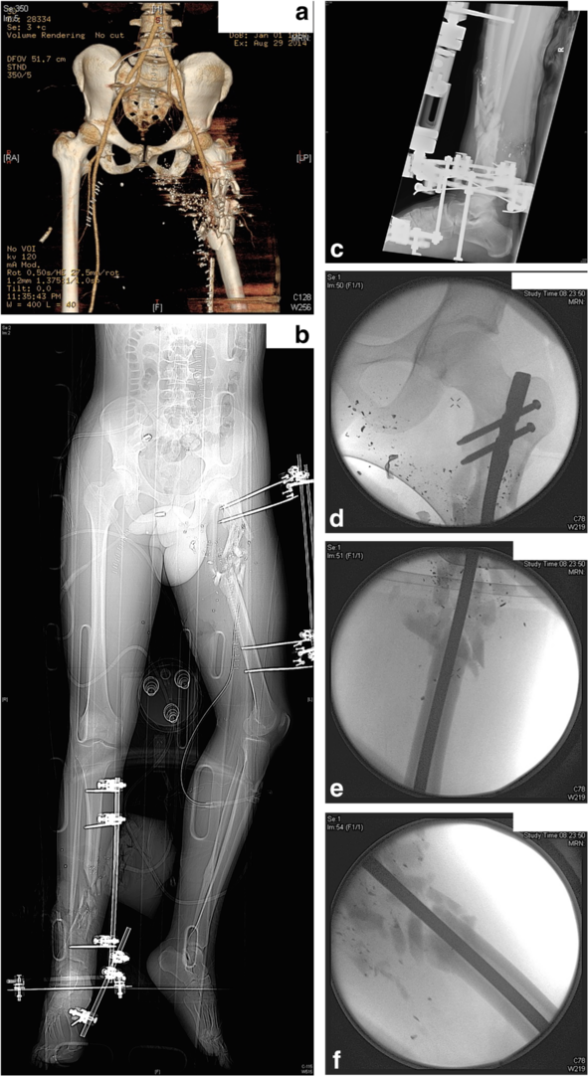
a 3D reconstruction showing extent of left comminuted open proximal femur injury. b Scout radiograph showing the original DCO procedures to the right tibia and left femur as described in the text. c Post-operative view of the right tibia after conversion to a hybrid ring fixator. d-f Intra-operative radiographs showing the comminuted subtrochanteric femoral fracture initially treated with external fixation but then converted definitively to an intramedullary nail
